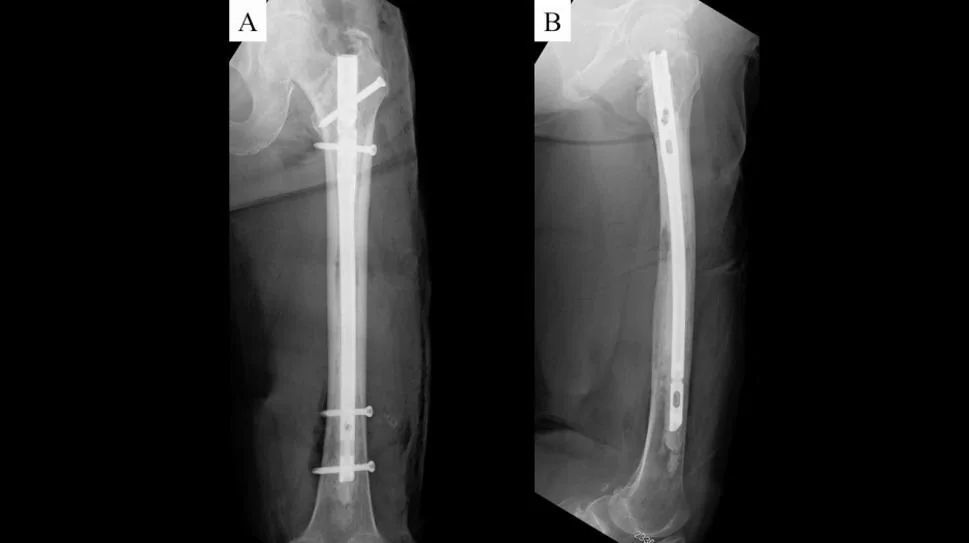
Postoperative radiographs after cement gun–assisted intramedullary nailing (CG-IMN). A Representative anteroposterior radiograph of the femur after CG-IMN. B Representative lateral radiograph of the femur after CG-IMN
